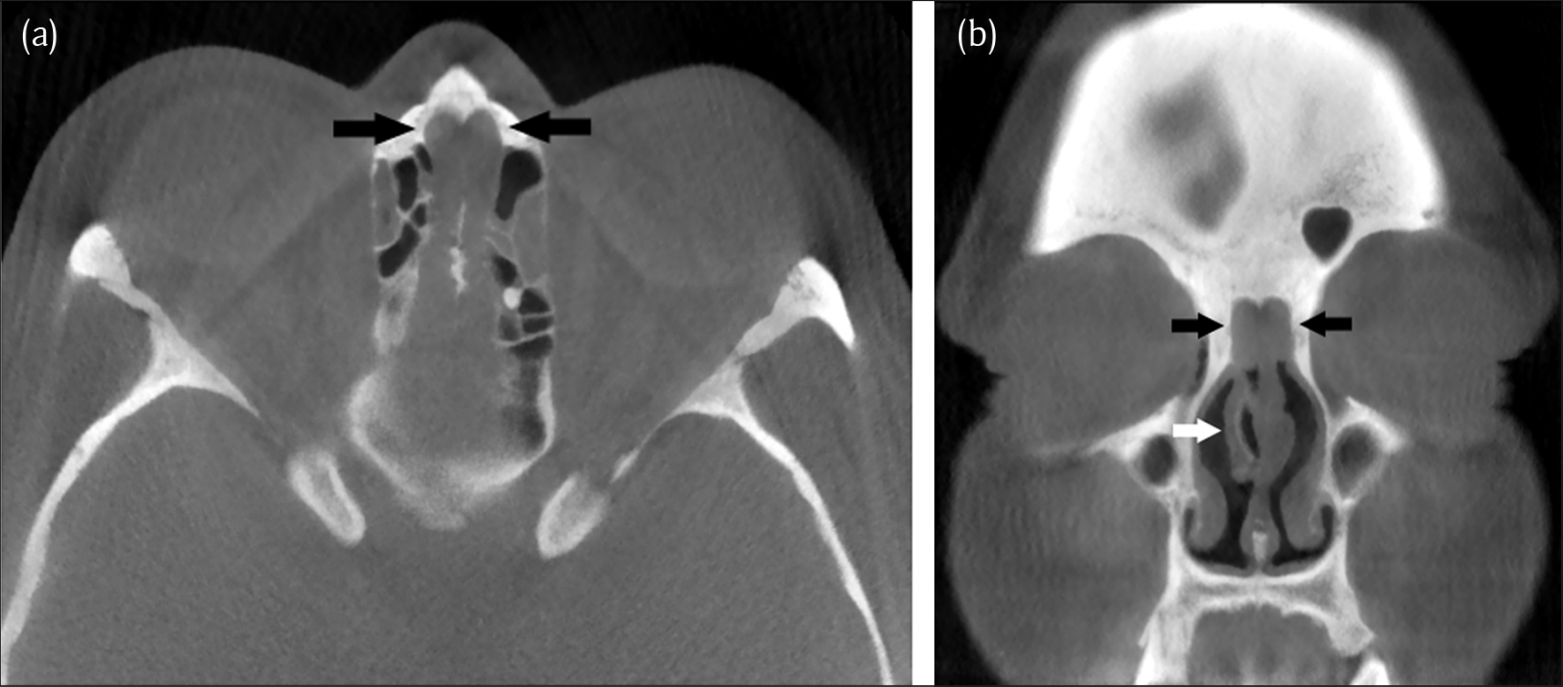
Axial (a) and coronal (b) CT images in bone window settings demonstrate the typical imaging findings. Bilateral opacification of the olfactory cleft leading to a total width of 1 cm (distance between the black arrows). The sharply delineated soft-tissue mass (black arrows) originate anteriorly and superiorly from the middle concha (white arrow). There is bone remodelling but no bone erosion.
